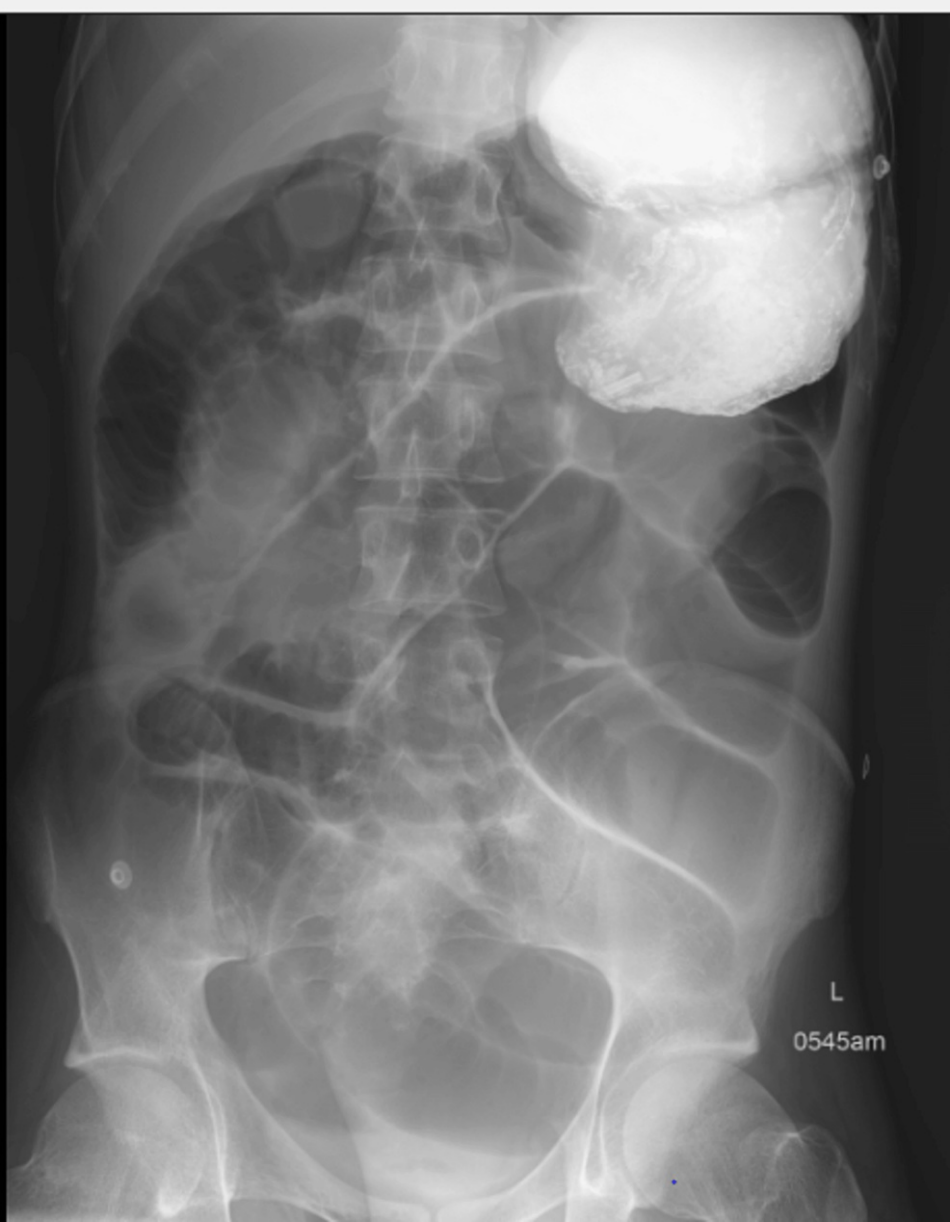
Gastrograffin X-ray of the abdomen study showing pooled contrast in the markedly dilated stomach and dilated colon loops
